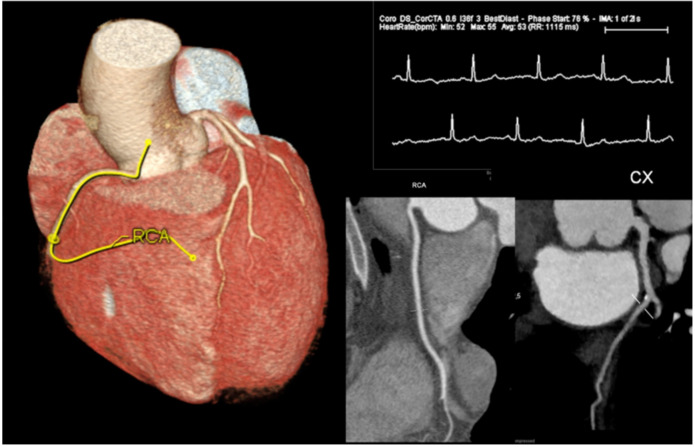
A 48-year-old male with intermediate risk of CAD and atypical chest pain. Cardiac gated CT showed no coronary stenosis, and the patient was safely discharged.
